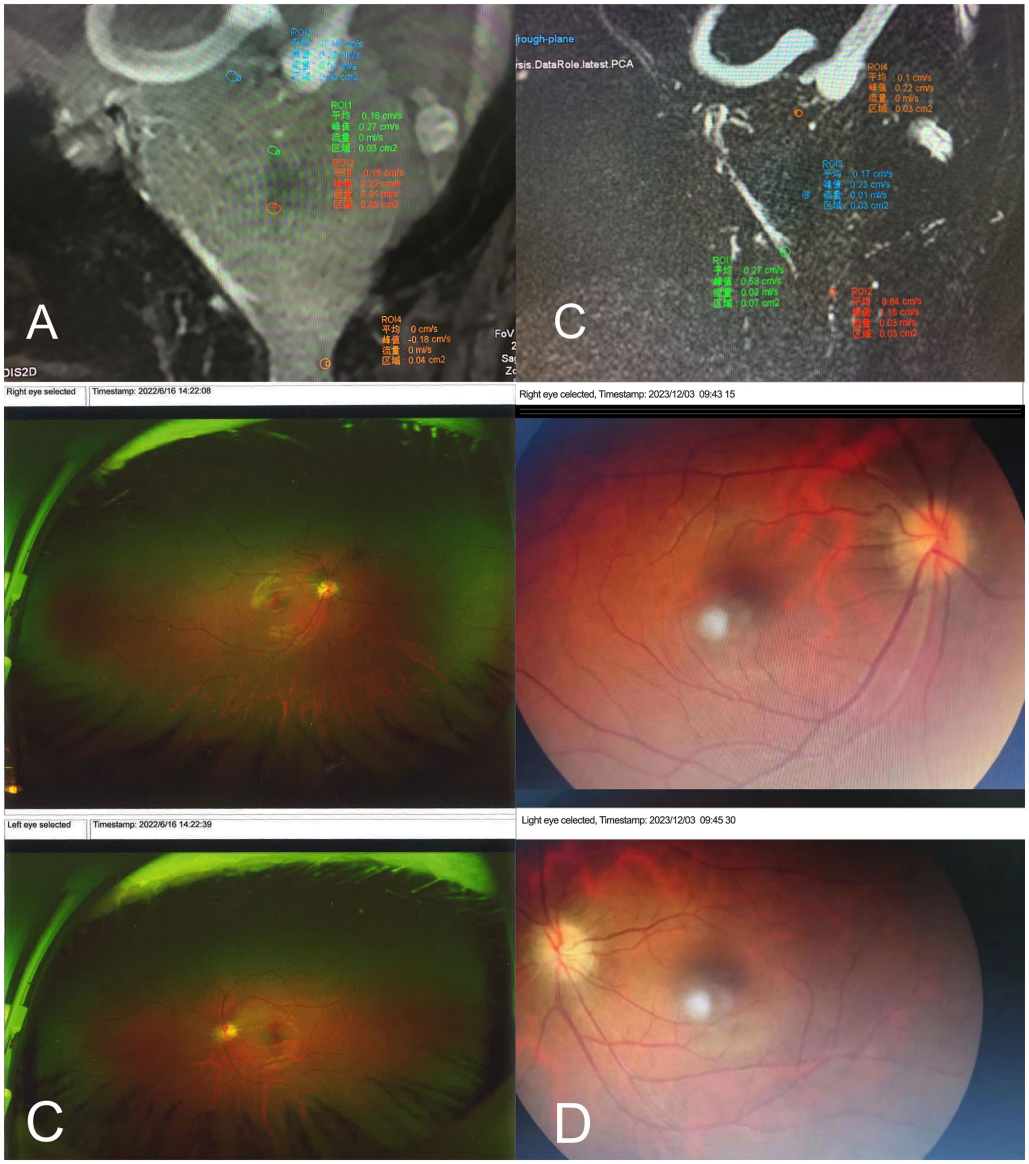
(A,B) The patient’s Cranial MRI with 2D-Q-FLOW sequence preoperatively measured the patient’s CVJ cerebrospinal fluid flow velocity in the mid-sagittal position. (A) the CSF flow velocity preoperatively in June 2022. It demonstrated an accelerated mean flow velocity in the aqueduct of sylvius (mean flow = 0.01 mL/s), the entrance to the four ventricles (mean flow = 0.00 mL/s), and the exit from the four ventricles (mean flow = 0.01 mL/s). Additionally, the flow area and total flow at the exit from the four ventricles are significantly reduced, as is the dorsal flow velocity in the craniocervical junction (mean flow = 0 mL/s). (B) CSF flow velocity postoperatively in December 2023. It demonstrated a slow mean flow velocity in the aqueduct of sylvius (mean flow = 0.0 mL/s), the entrance to the cisterna magna (mean flow = 0.01 mL/s), the exit from the quadrigeminal ventricles (mean flow = 0.02 mL/s), and recovery of the dorsal flow velocity in the craniocervical junction area compared to before (mean flow = 0.03 mL/s). (C,D) The patient’s results from ocular coherence tomography (OCT) examination. (C) Preoperative OCT revealed the presence of bilateral severe papilledema. (D)The 18 months postoperatively, and the severe papilledema was completely relieved.
